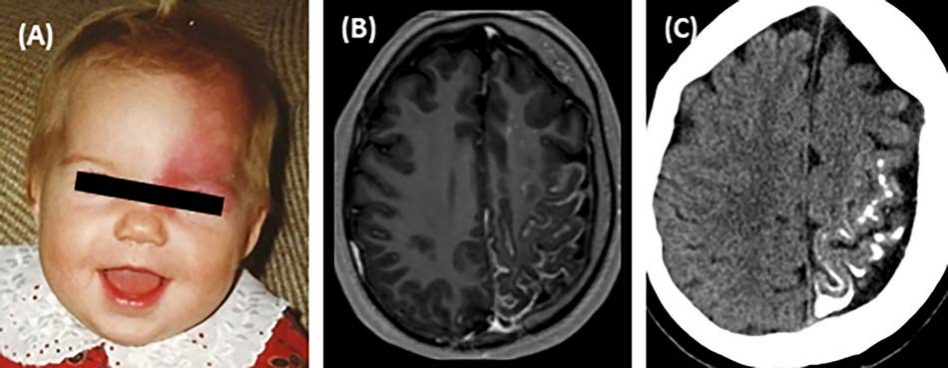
Clinical features of patient 3. (A) Childhood image of the patient displaying a port-wine lesion affecting her left eyelid and brow. (B) Contrast-enhanced MRI shows left-sided enhancement in leptomeningeal vascular malformation and atrophy. (C) Non-contrast-enhanced CT shows gyral calcifications and atrophy.
